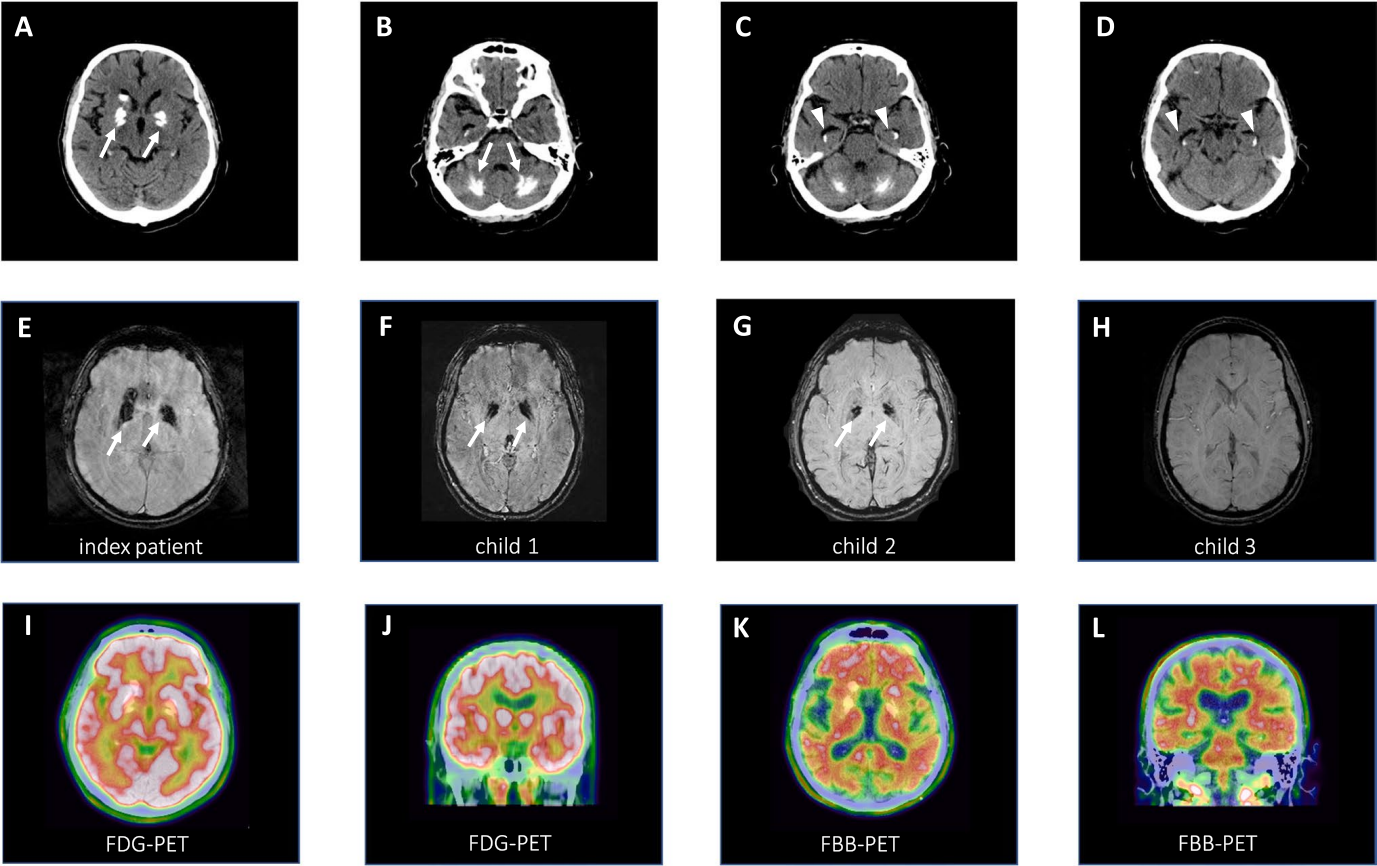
Axial cerebral CT and susceptibility-weighted MR imaging of the index patient (A–E) shows extensive calcifications of bilateral basal ganglia (in pallidum and caudate nucleus) as well as in both cerebellar hemispheres (arrows) and bilateral hippocampus (arrowheads). Susceptibility-weighted MR imaging of the index patient’s children (F–H) shows symmetrical calcifications of the basal ganglia (arrows) in both child 1 and child 2. Child 3 shows no calcifications. Axial and coronal FDG-PET/CT (I, J) of the index patient demonstrates areas of slightly reduced glucose metabolism in parietal and mesiotemporal cortex. FBB-PET/CT (K, L) study in axial and coronal sections shows widespread β-amyloid deposits in the frontal and temporal cortex but also in the parietal cortex. Red/light red indicates high values; green/blue indicates low values
